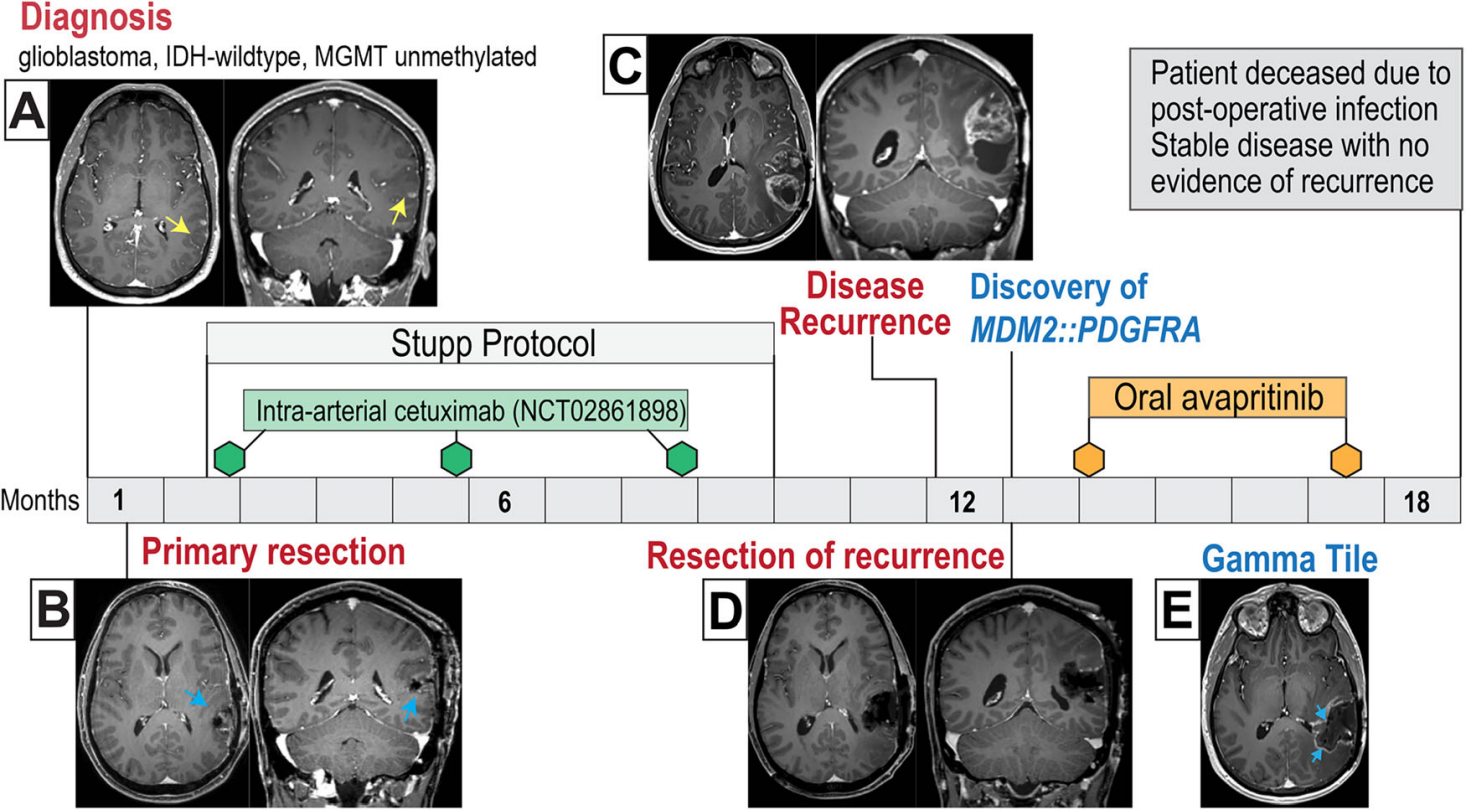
Patient’s clinical course with radiographic findings. A Initial presentation, axial and coronal T1 MRI images demonstrate T2 expansile lesion in the left posterior superior temporal gyrus with small area of enhancement near the superior temporal sulcus. B Post-operative axial and coronal T1 scan following initial resection demonstrating gross total resection. C Post-gadolinium axial and coronal T1 images reveal disease progression with a new cystic mass with peripheral enhancement in the left temporal lobe. D Post-operative axial and coronal T1 scan following second resection demonstrating gross total resection. E Axial T1 images demonstrate correct intraoperative placement of GammaTile brachytherapy.
